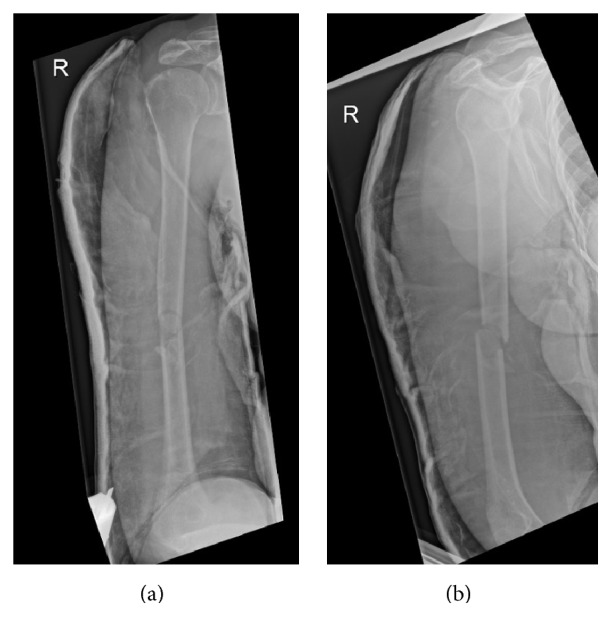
Post-reduction radiographs demonstrating excellent alignment.
